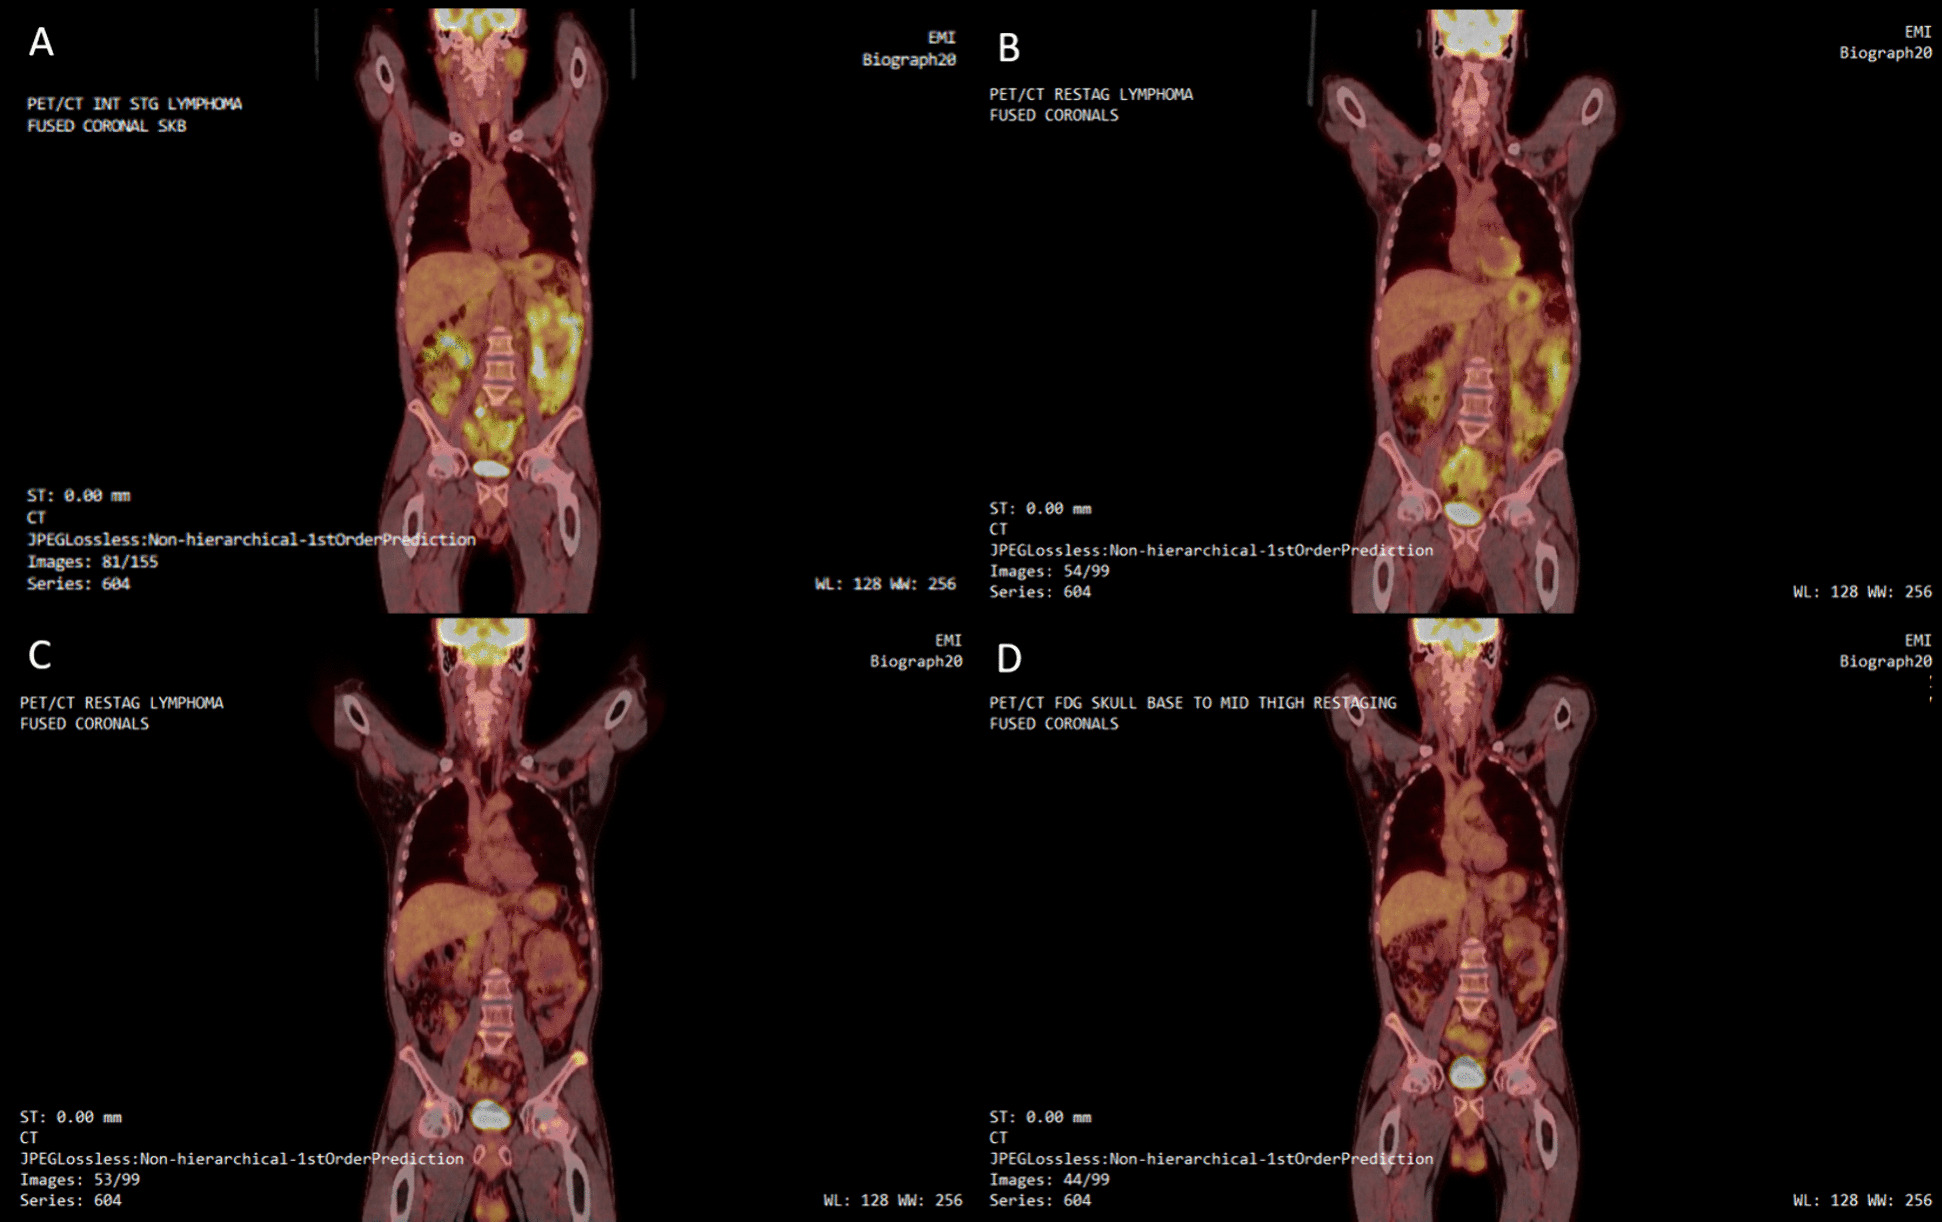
F-18 fluorodeoxyglucose (^18^F-FDG) positron emission tomography (PET) and/or computed tomography (CT) images: A before fasting; B two months after first fast; C five months after second fast; D nine months after second fast occurring over a span of two years
